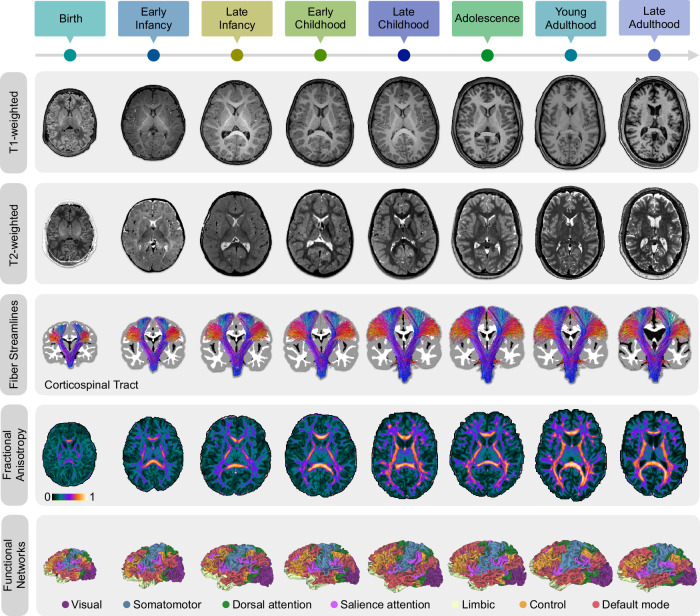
Multiscale brain changes across the human lifespan. T1-weighted and T2-weighted brain MRIs, white matter tracts, fractional anisotropy, and large-scale functional networks of typically-developing individuals between birth and advanced age.
